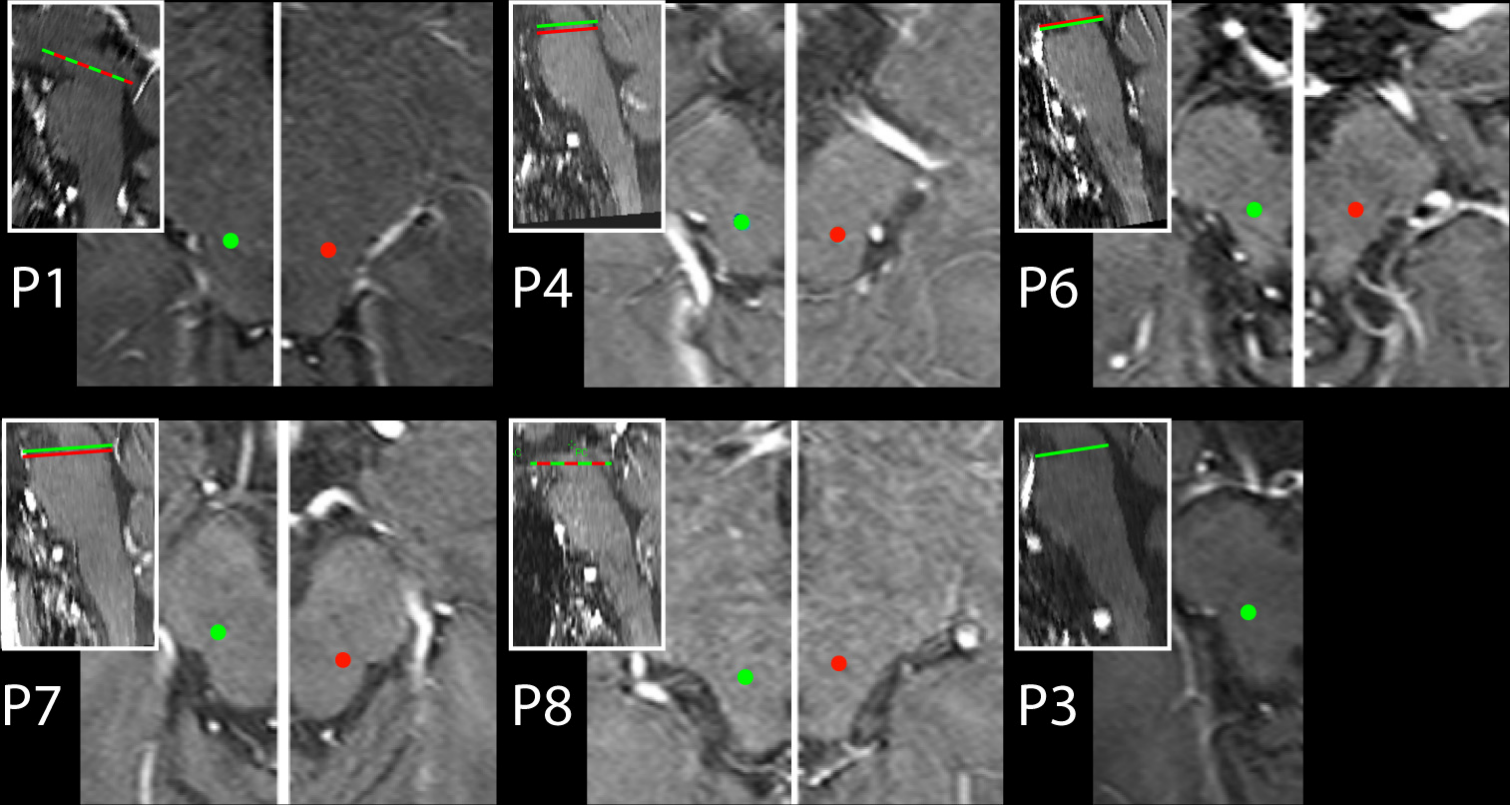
DBS electrode contact locations. The location of the active electrode contacts in the right (green dots) and left (red dots) PPN region. Shown are axial MRI transsections in each patient. Left and right side transsections display different planes along the rostro-caudal axis in P4, P6, and P7. Respective planes are highlighted by green (right side) and red lines (left side) in the inserts showing midline sagittal transsections.
